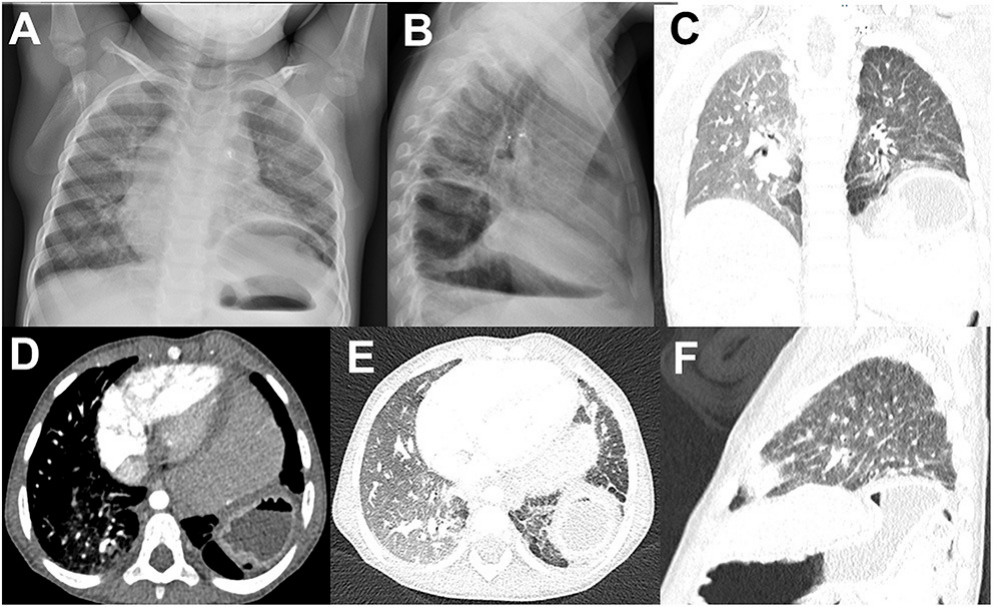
Radiological image of the major recurrence of left side CDH after a first patched diaphragmatic closure. (A,B) Chest X-ray image, (C–F) computed tomography image.
